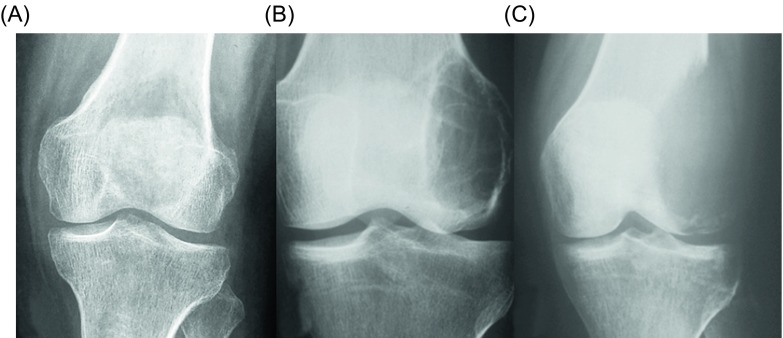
Campanacci et al. [3] grading system for GCT that is based on the radiographic appearance of the tumors. (A) Grade 1 (latent), (B) grade 2 (active), and (C) grade 3 (aggressive).
